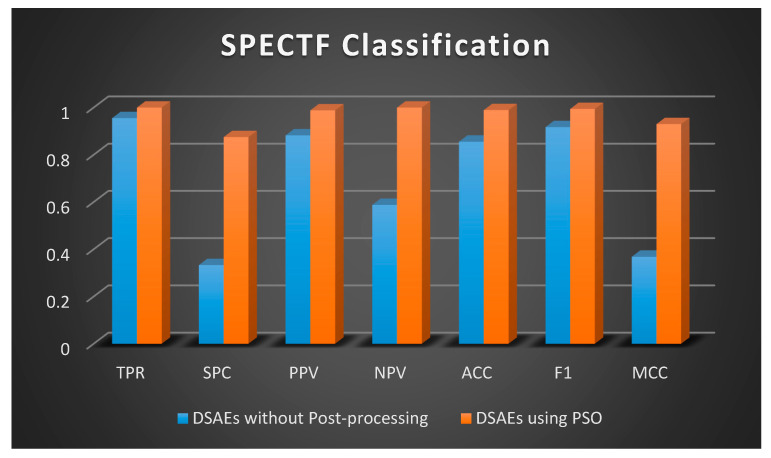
Graphical Representation of Performance Criteria for SPECTF Classification.
